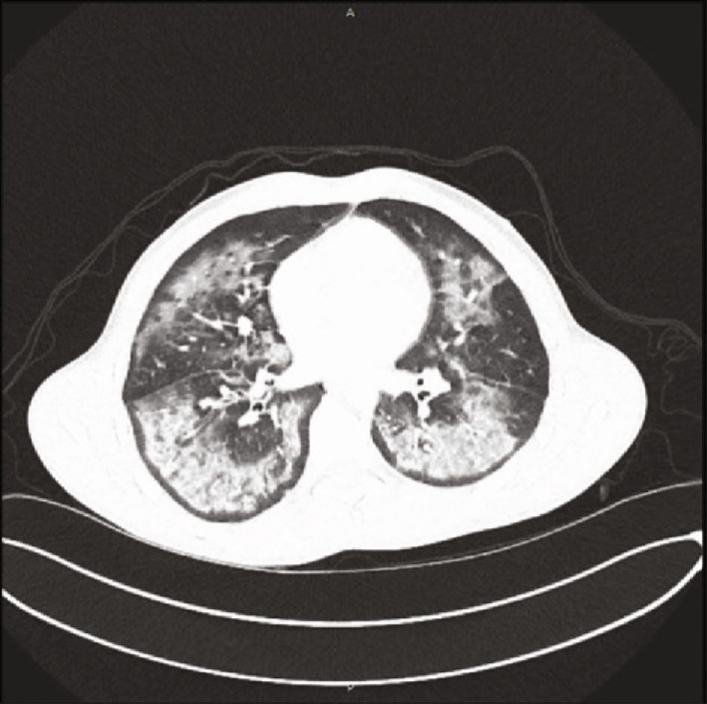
Case 2 chest CT scan on presentation (axial view).
